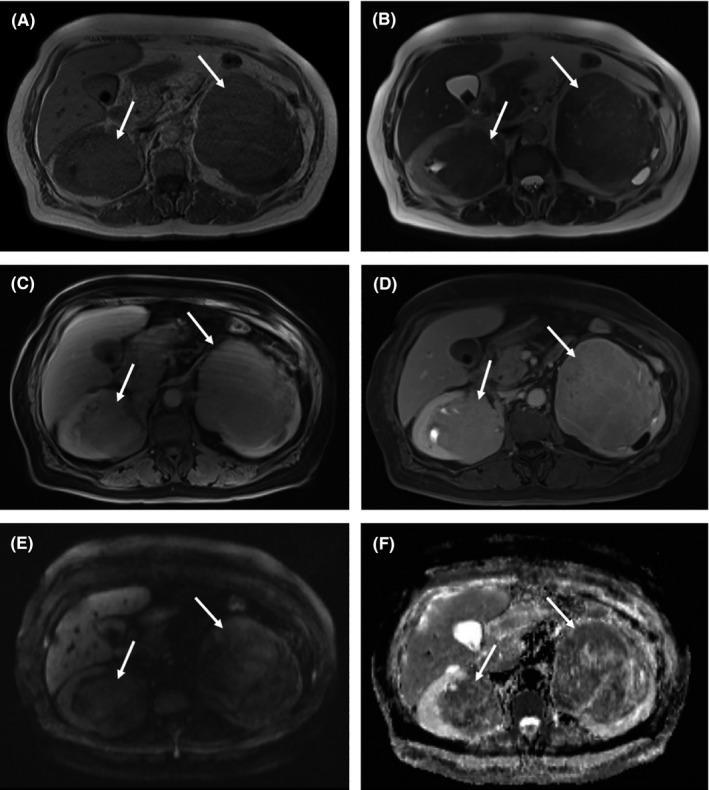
A‐F, Magnetic resonance imaging (MRI) of bilateral renal masses in a patient with Rosai‐Dorfman disease. A, Masses (*thin arrows*) have hypo‐to isointense signal on transverse T1‐weighted image and B, appear as mild heterogeneous hyperintense masses on transverse T2‐weighted image C, Masses have moderate enhancement on transverse gadoxetic acid‐enhanced nephrogenic phase image and D, sustain enhancement in the delayed phase. E, Mild hyperintense masses on diffusion‐weighted imaging obtained with a b‐value of 800 s/mm^2^ and F, hypointense masses on apparent diffusion coefficient map
